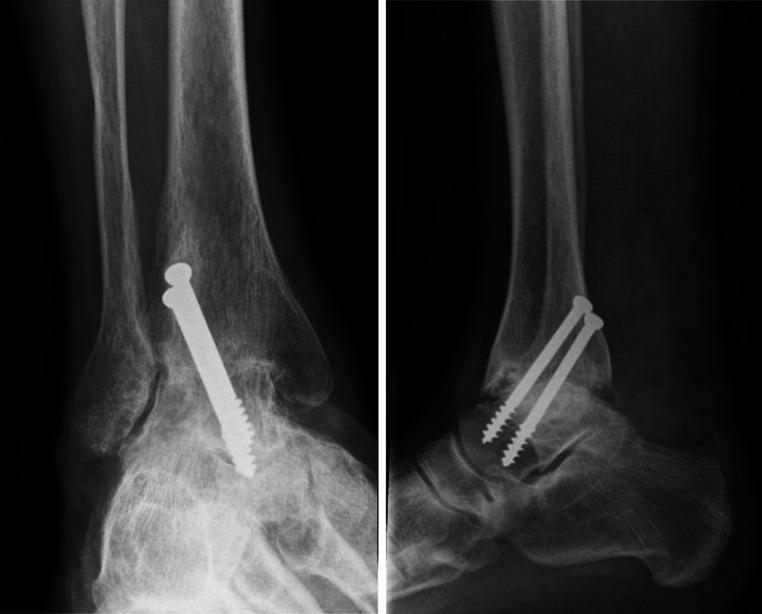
Weight-bearing radiographs in the anterior-to-posterior and lateral direction at 1-year follow-up with union in both radiographic planes
